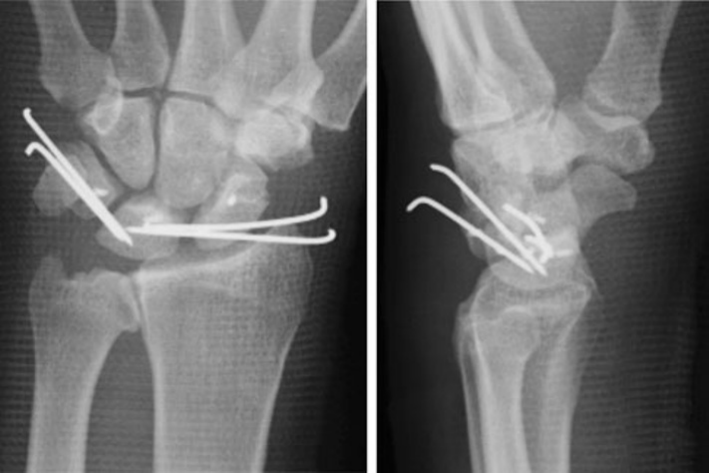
Postoperative radiographs showing good alignment of the carpal bones. The scapholunate angle was 54° and the radiolunate angle 6°. Gilula’s line was well-regulated
